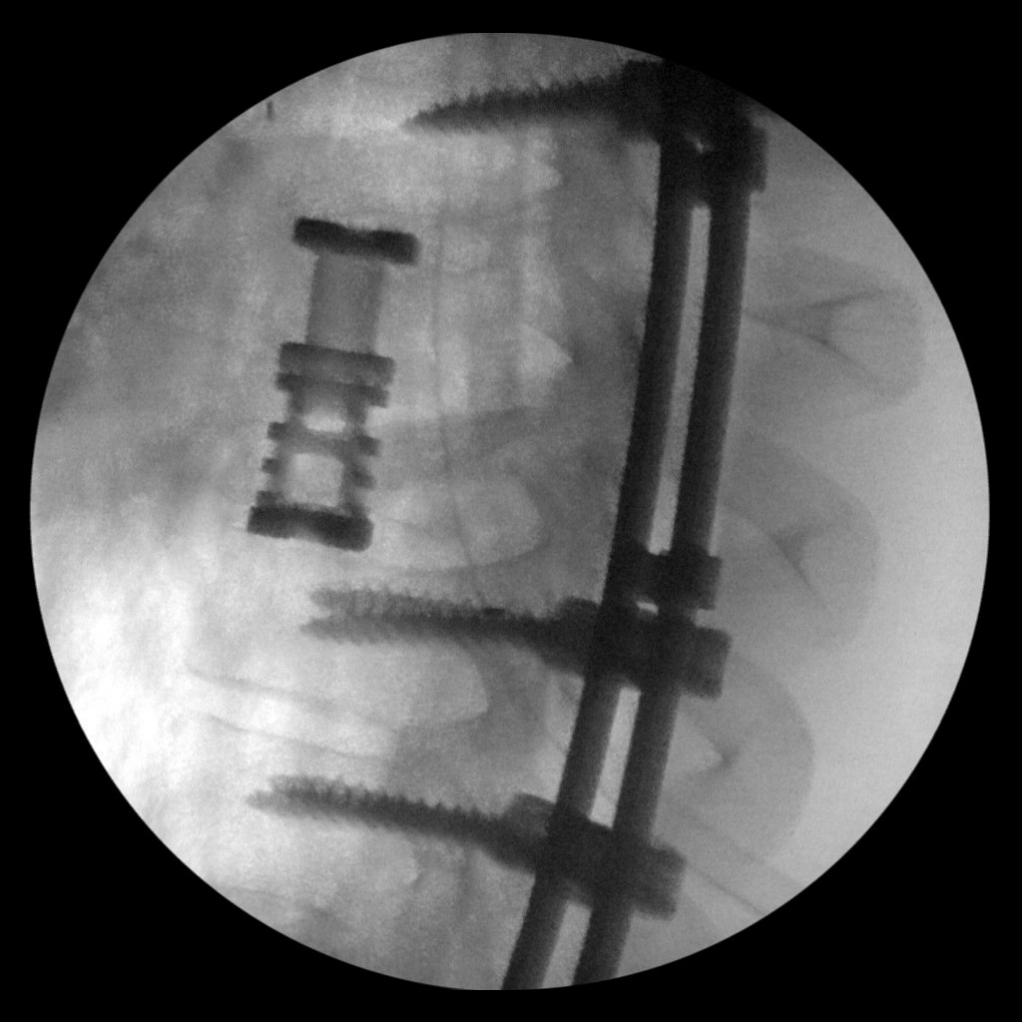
Postoperative lateral radiograph showing placement of T7-8 cage
